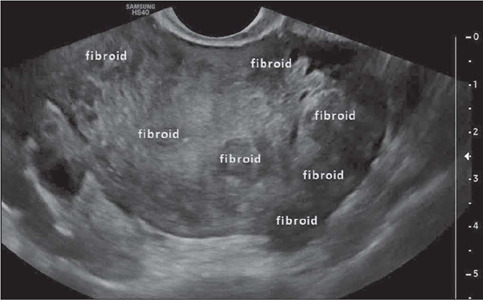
Transvaginal ultrasound image, in a cross-sectional view, showing myomatosis in a
